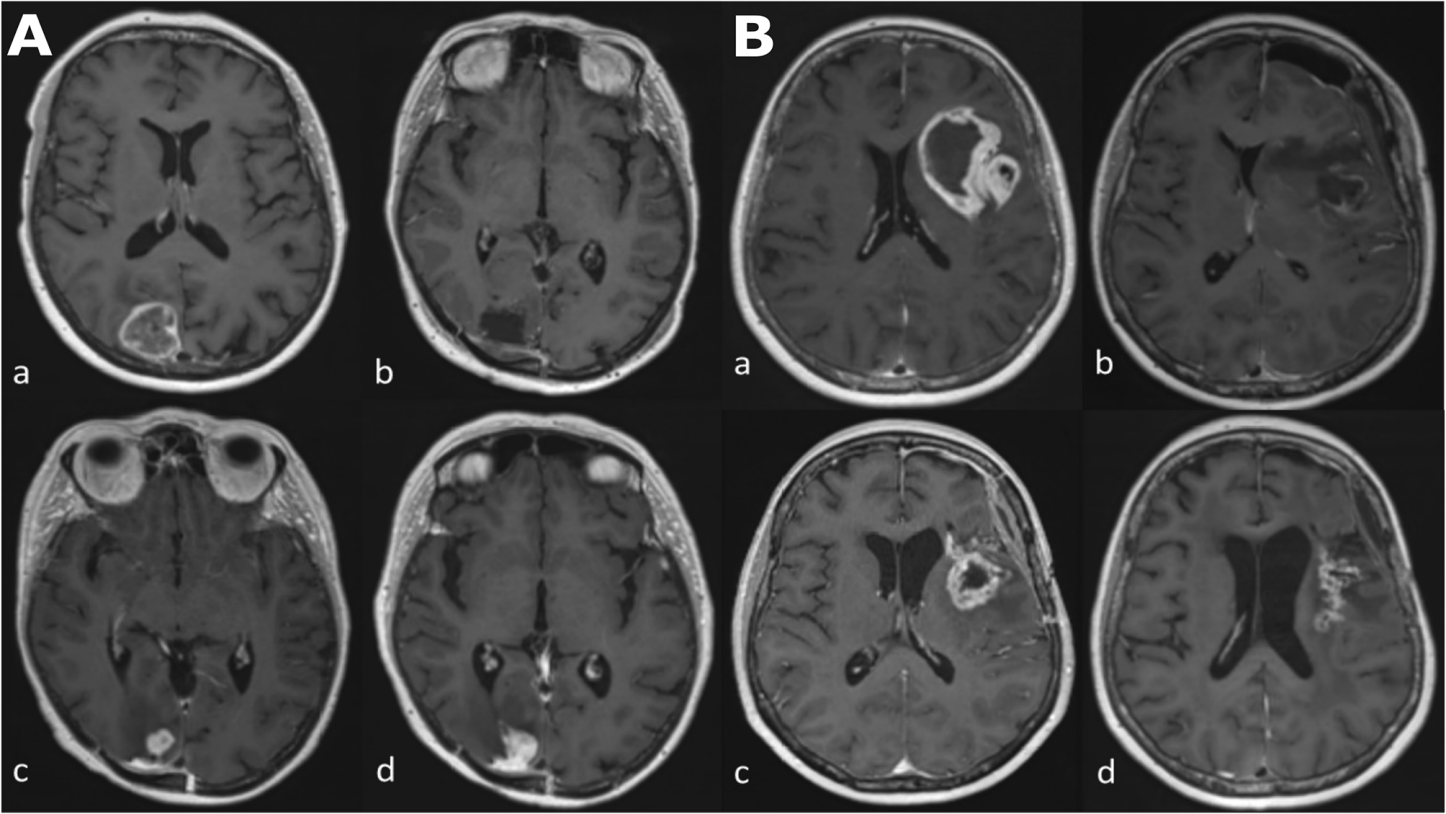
Longitudinal series of MRI images in two patients (A, B) with glioblastoma, IDH-wildtype. All images are axial *T*_1_-weighted after contrast administration. Images (Aa–Ad) demonstrate tumor progression. (Aa) Pre-operative MRI of a glioblastoma in the occipital lobe. (Ab) Post-operative MRI five days after resection; there is no contrast enhancement therefore no identifiable residual tumor. (Ac) The patient underwent a standard care regimen of radiotherapy and temozolomide. A new enhancing lesion at the inferior margin of the post-operative cavity was identified on MRI at three months after radiotherapy completion. (Ad) The enhancing lesion continued to increase in size three months later and was confirmed to represent tumor recurrence after repeat surgery. Images (Ba–Bd) demonstrate pseudoprogression. (Ba) Pre-operative MRI of a glioblastoma in the insula lobe. (Bb) Post-operative MRI at 24 hours after surgery; post-operative blood products are present but there is no contrast enhancement therefore no identifiable residual tumor. (Bc) The patient underwent a standard care regimen of radiotherapy and temozolomide. A new rim-enhancing lesion was present on MRI at five months after radiotherapy completion. (Bd) Follow-up MRI at monthly intervals showed a gradual reduction in the size of the rim-enhancing lesion without any change in the standard care regimen of radiotherapy and temozolomide or corticosteroid use. The image shown here is the MRI four months later.
